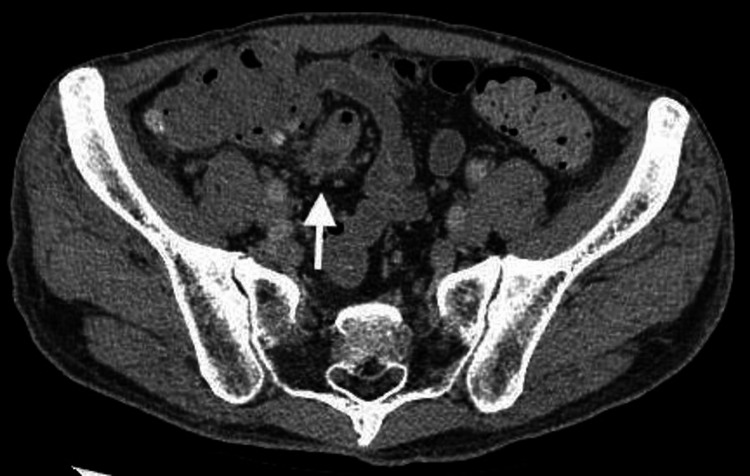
Approximately 2 cm abscess (arrow) This corresponds to the location indicated by the arrow in Figure 2, which could not be identified as an abscess on the plain CT.
